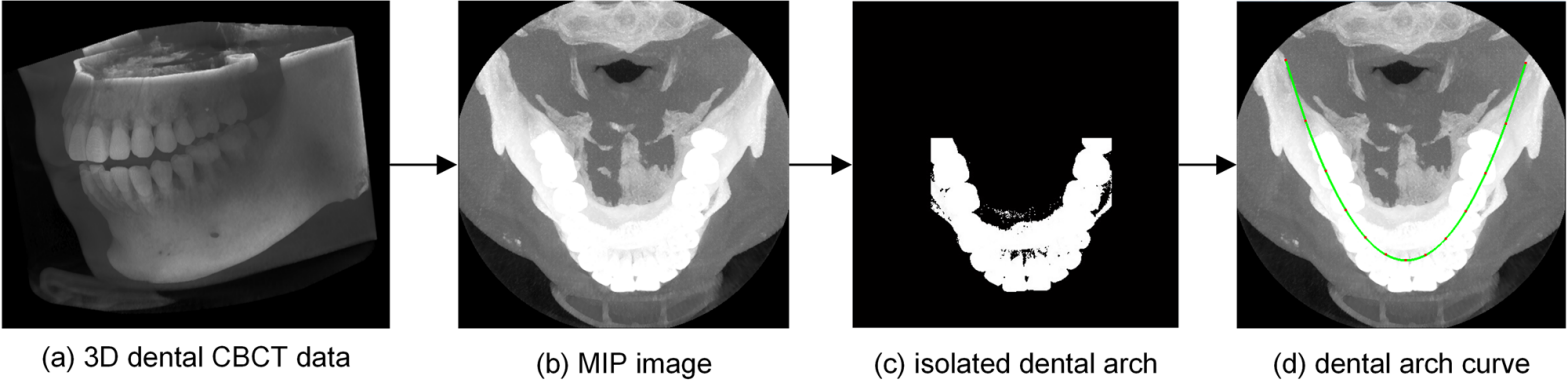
Visualization of the dental arch curve creation applied to a 3D dental CBCT data.
